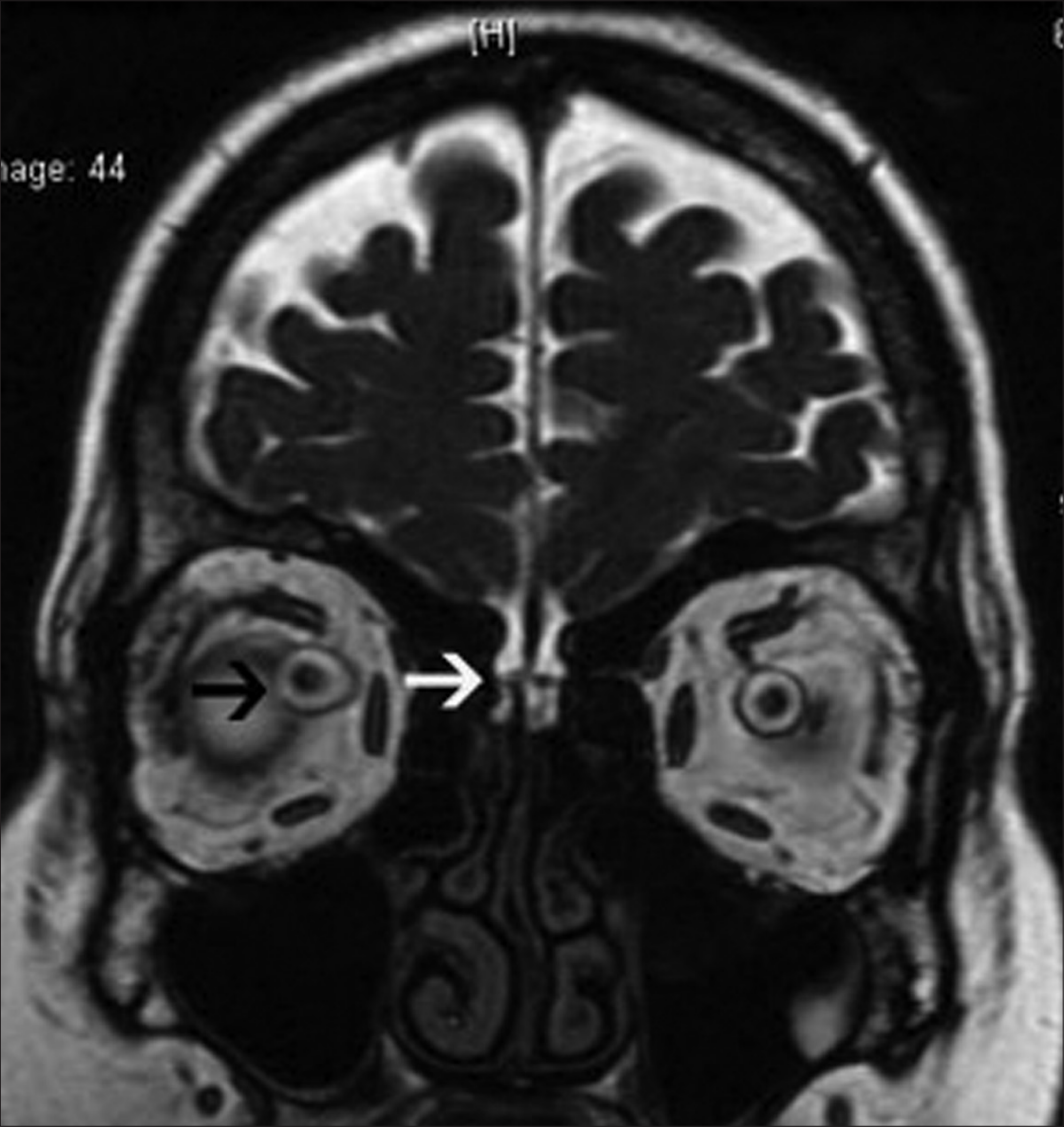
The coronal T2-weighted image reveals a leak in the cribriform plate into the anterior ethmoid cells (white arrow) and distension of the perioptic subarachnoid space (black arrow)
